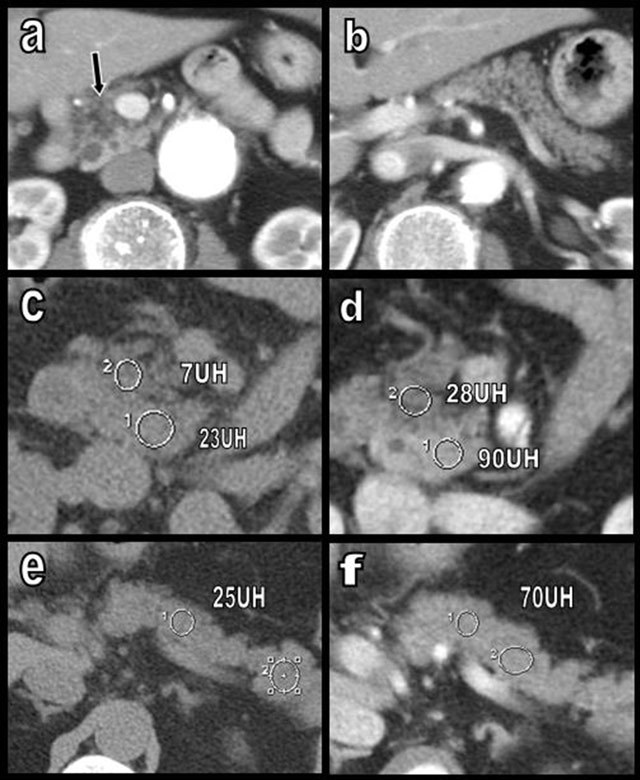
Typical case of uneven FI (a and b) limited to the APH (type 1a) (black arrow). However, the typical “negative” density of fat is not so obvious because the measures of density invariably include residual glandular acini. These acini also enhance with intravenous contrast of the rest of the gland. Nevertheless, the global contrast enhancement of the lipomatous area (from 7 UH (c) to 28 UH (d)) remains inferior to that of the rest of the gland (from 23–25 UH (e) to 70–90 UH (f)). This situation may mimic a pancreatic cancer, especially when the signal-to-noise ratio of the image is poor or if the slice thickness is not appropriated. In difficult or ambiguous cases, ultrasound or MRI can help in the differential diagnosis.
